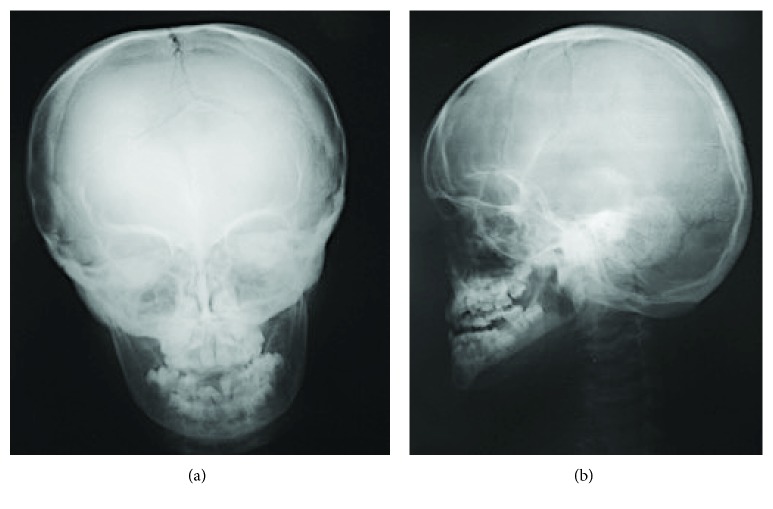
(a, b) Delayed closure of the sutures, widening of the anterior fontanelle, Wormian bones, sclerotic skull base, numerous supernumerary teeth, and malocclusion can be seen in the cranial radiographs (11 years).
